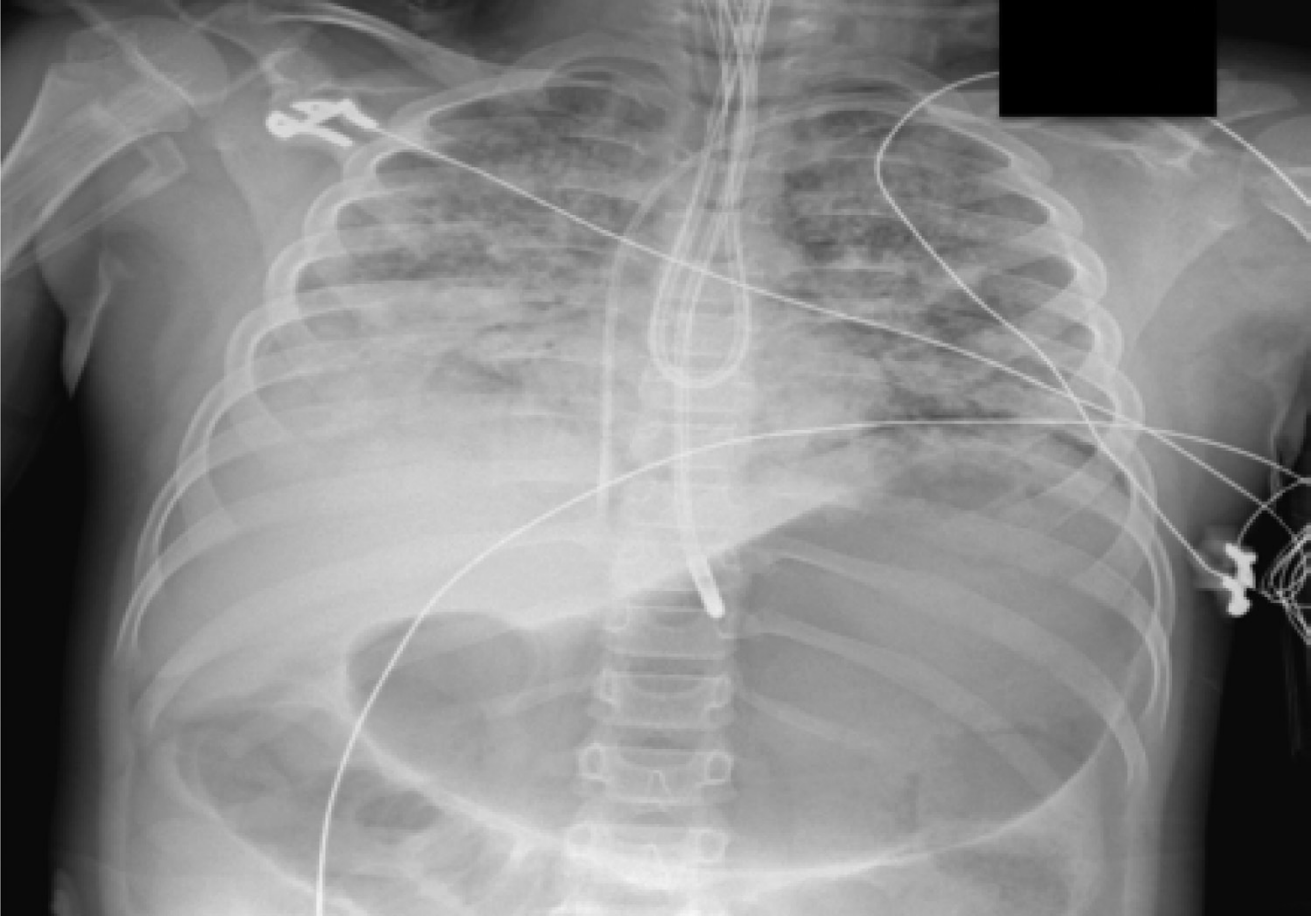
Chest and abdominal x-ray with the nasogastric tube unable to be advanced past the distal esophagus with hyperinflated gastric bubble.
